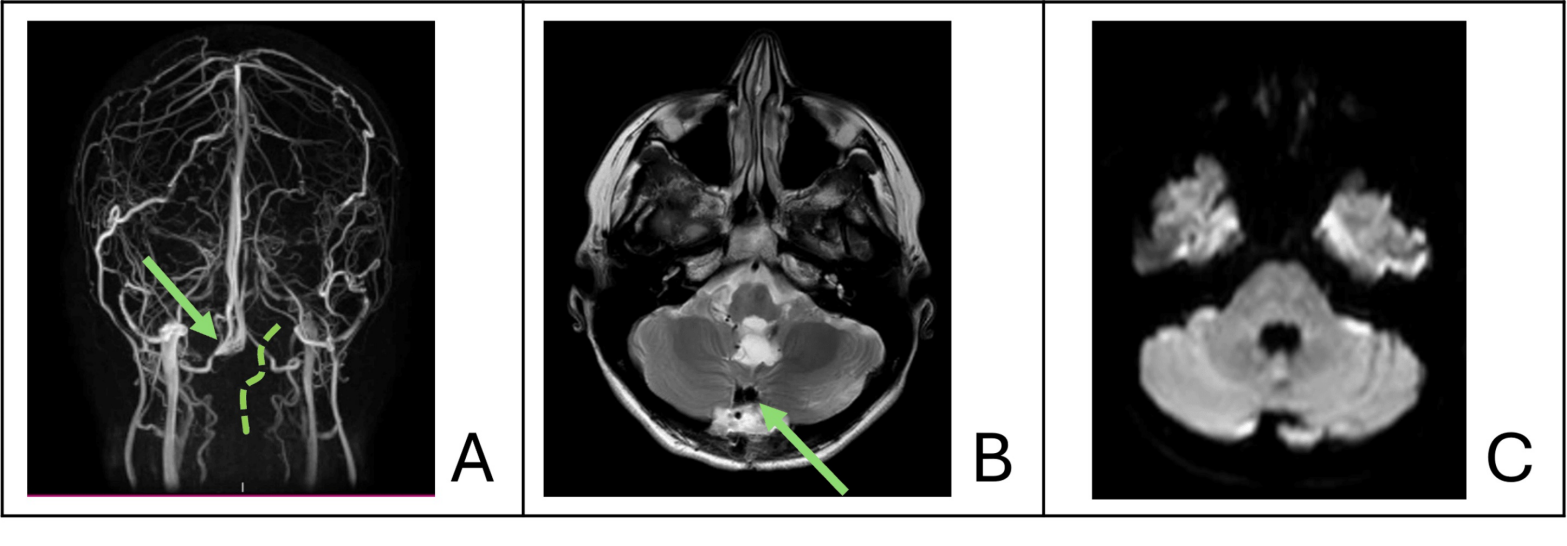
Postoperative magnetic resonance imaging (A) Coronal MR venography showing patent dominant occipital and right marginal sinuses (arrow) and ligation of left marginal sinus at site of paramedian durotomy (dotted line); (B) Axial T2 MRI showing gross total resection with patent occipital sinus flow void (arrow); (C) Axial diffusion-weighted image showing no evidence of residual epidermoid tumour
